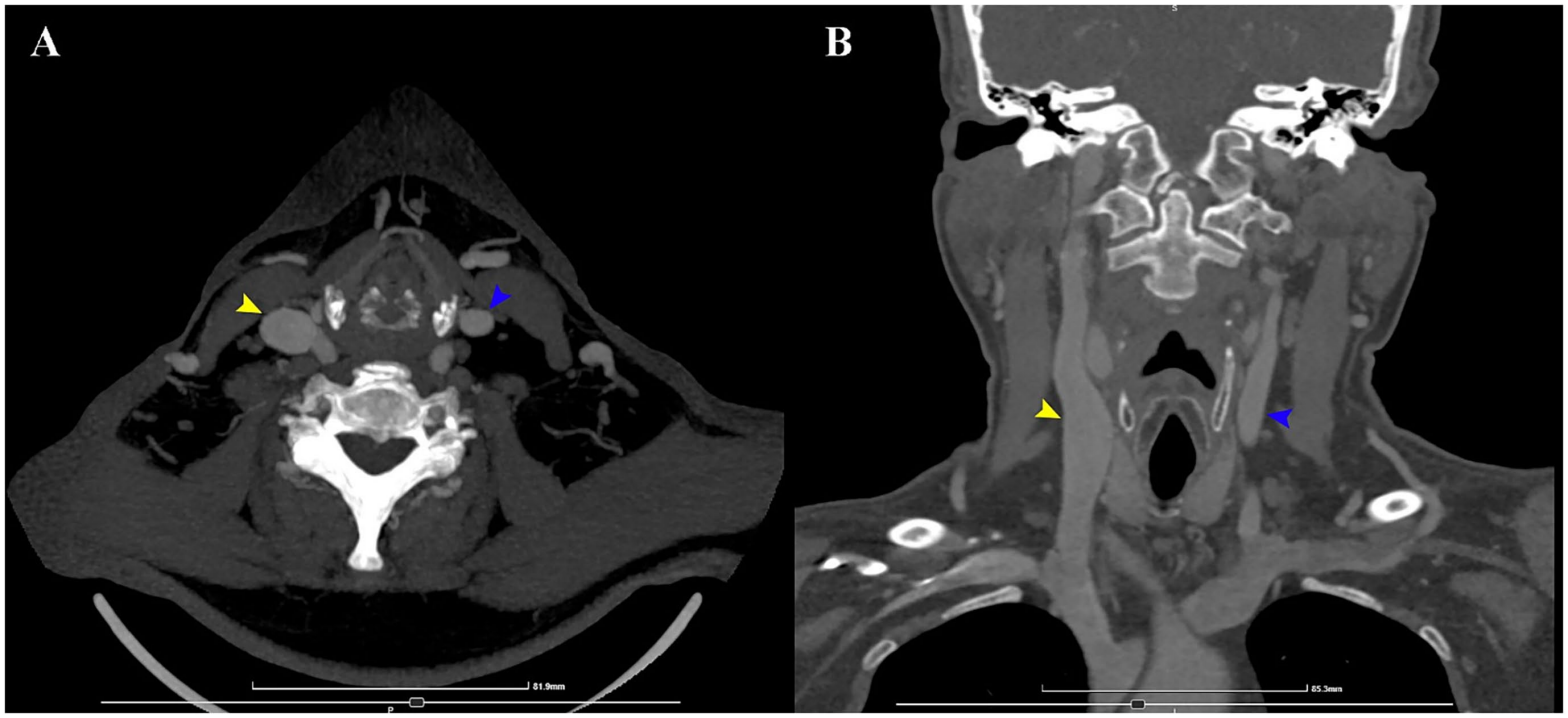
CT angiography of the neck axial (A) and coronal (B) sections. The internal jugular veins are visible (yellow, respectively blue arrows). The maximum transverse and anteroposterior diameters of the internal jugular veins were 1.53 cm and 1.75 cm on the right, and 0.87 cm and 0.72 cm on the left, respectively. The corresponding cross-sectional areas were approximately 2.11 cm^2^ on the right and 0.50 cm^2^ on the left.
